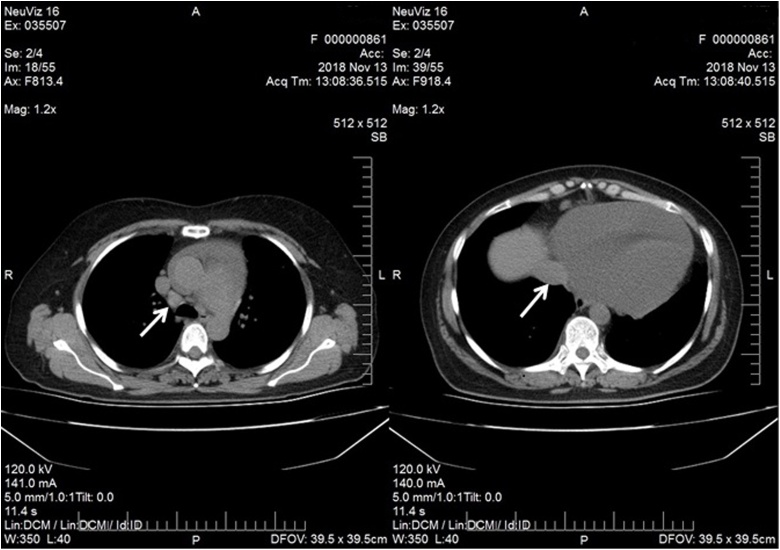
Computed tomography images of lymph nodes enlargement (arrows) in anterior mediastinum.
